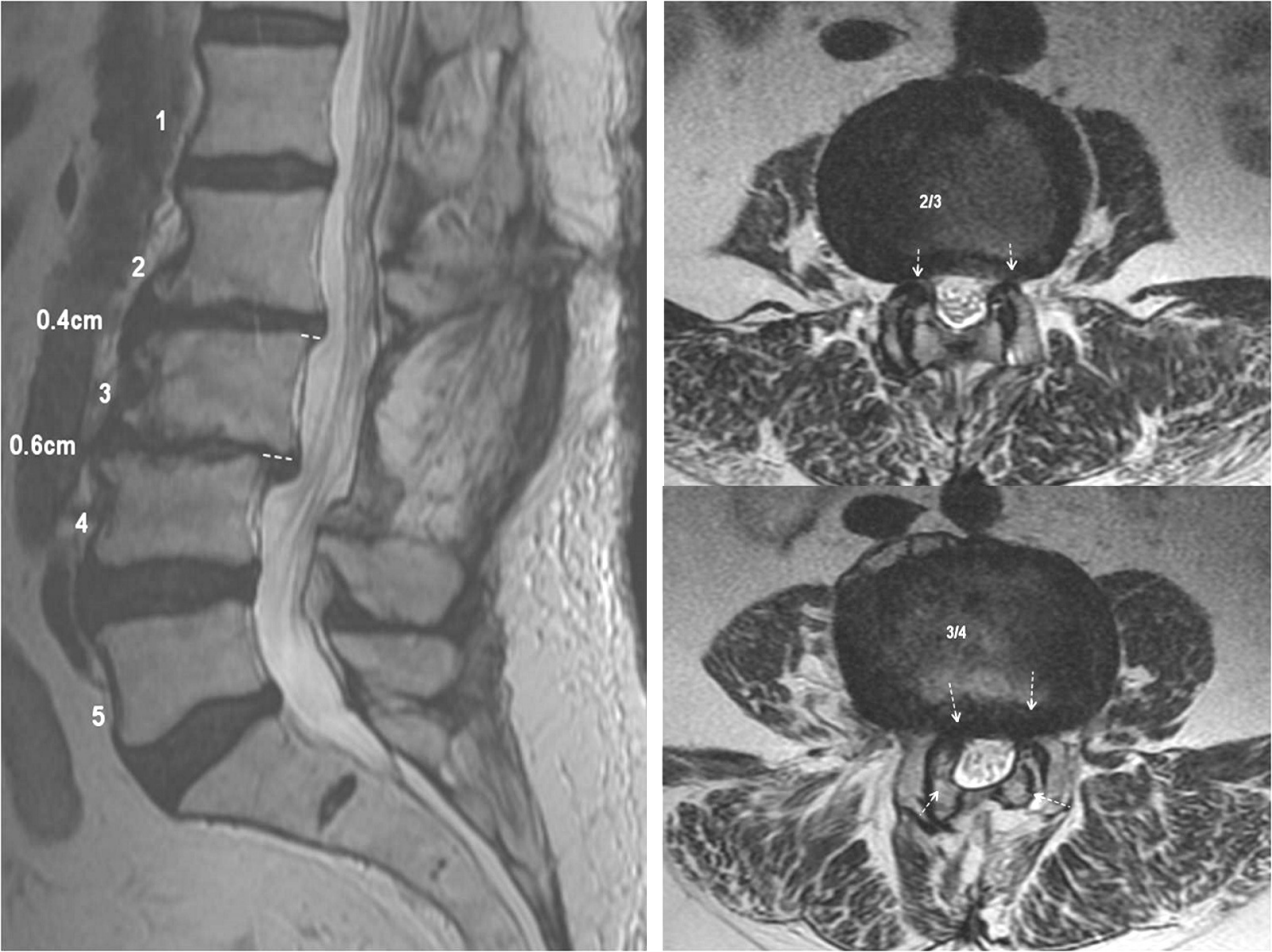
Preoperative MRI L2-3 and L3-4 degenerative disc disease, levoscoliosis, Grade 1 spondylolisthesis
